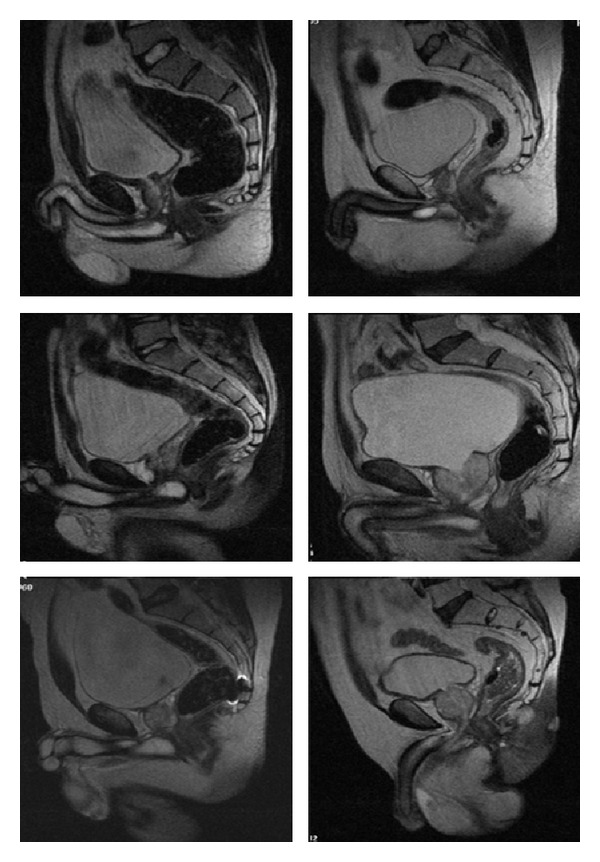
MRI images showing spheric and cone bladder shapes.
